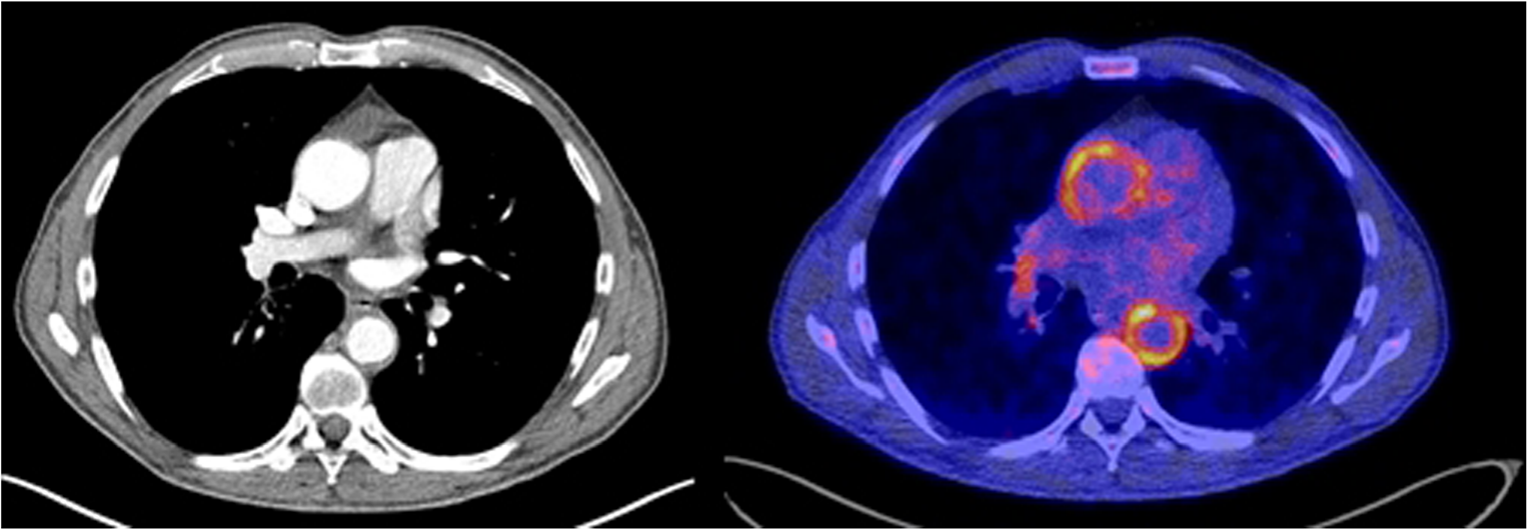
FDG-PET/CTA. On the left, a transaxial view of a contrast chest CT in a 67-year-old man with GCA, with an enlarged diameter of the ascending aorta of 41 × 41 mm and moderately increased wall thickness of 3.1 mm, and severely increased wall thickness of 4.7 mm of the descending aorta (diameter of 30 × 31 mm). On the right, the fused transaxial images of the contrast chest CT and FDG-PET showing highly elevated FDG uptake (average SUV_max_ 5.5) in the ascending and descending aorta
